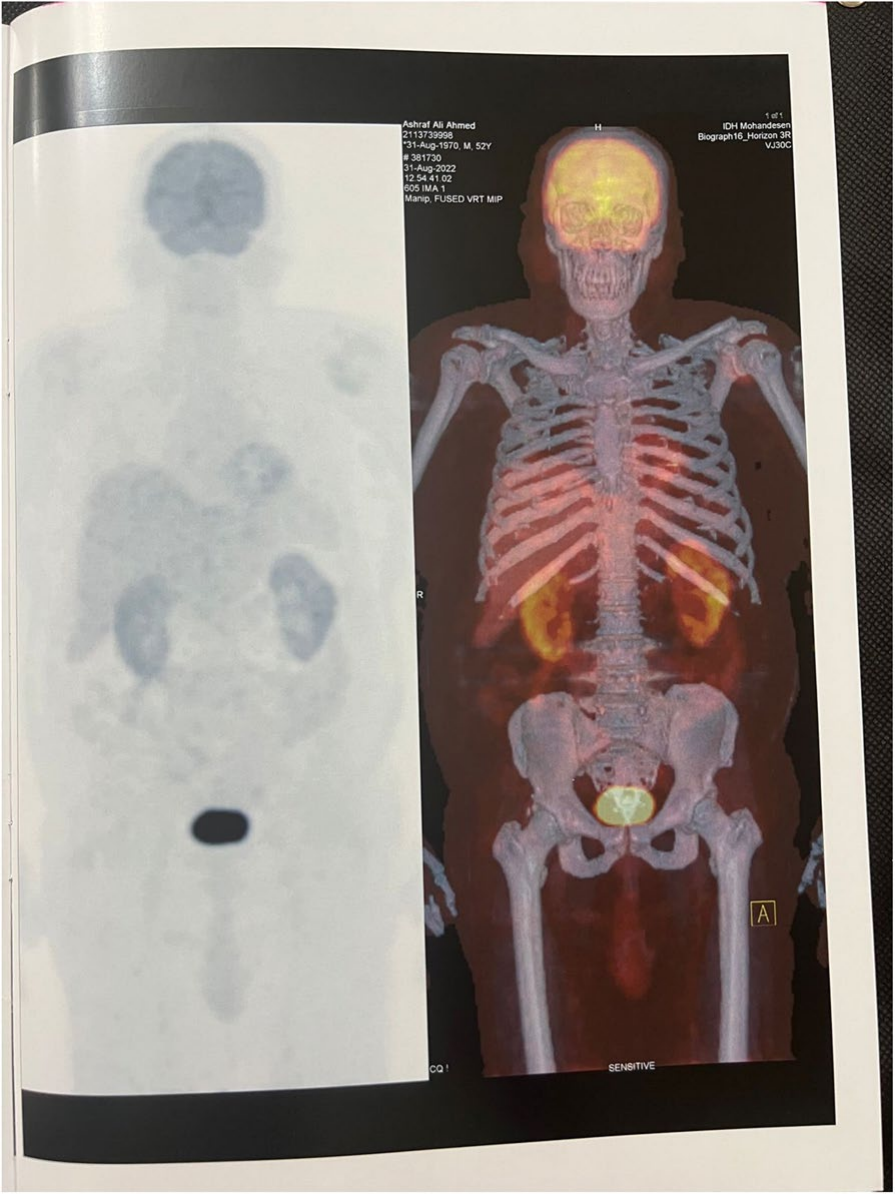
Posttreatment whole-body PET-CT. Morphological regression of the metabolically inert multiple hepatic focal lesions with no newly developed metabolically active lesions
